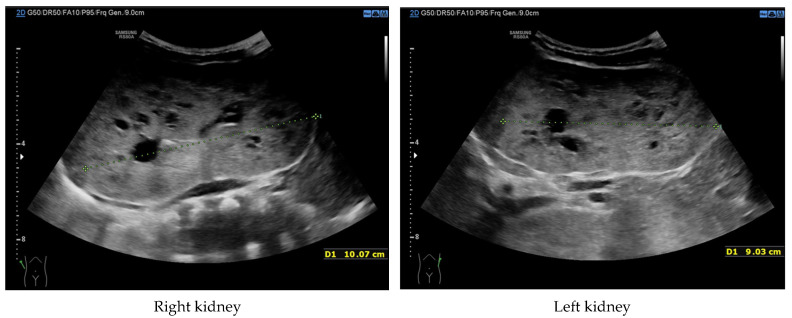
Ultrasonography of kidneys at the age of 4 months (enlarged kidneys with multiple cysts).
